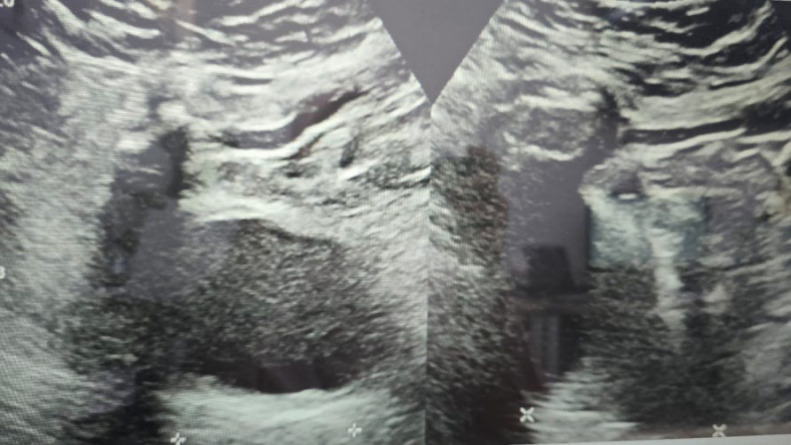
Ultrasound Examination. The uterus measured 3.89 × 4.86 cm, with no masses observed in either adnexa.
